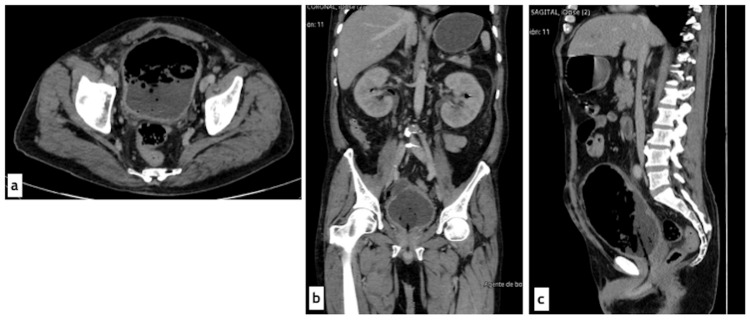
Abdominal computed tomography scan at admission. Contrast-enhanced abdominal computed tomography, venous phase: (a) Axial view at the pelvic cavity level showing a distended bladder with generalized wall thickening, intravesical sediment, and intravesical gas. (b) Coronal view at the renal level demonstrating emphysematous pyelonephritis (Huan-Tseng type IV), right-sided emphysematous pyelitis, bilateral ureteral dilation, and bladder findings similar to those described in (a). (c) Sagittal view showing marked bladder distention, urinary sediment, and abundant intravesical gas.
